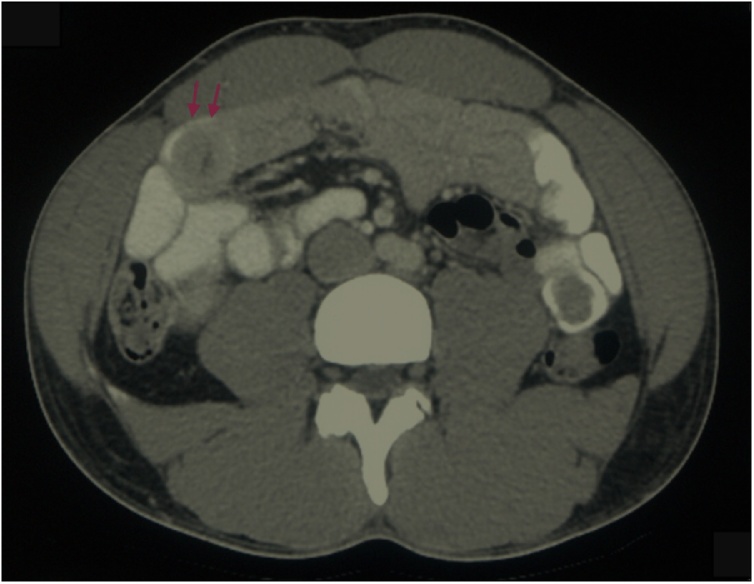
CT abdomen axial view demonstrating mild diffuse small bowel wall thickening, with two separate areas of apparent short segment jejunal intussusception without evidence of obstruction, and the characteristic ‘target sign’ indicative of intussusception (red arrows).
